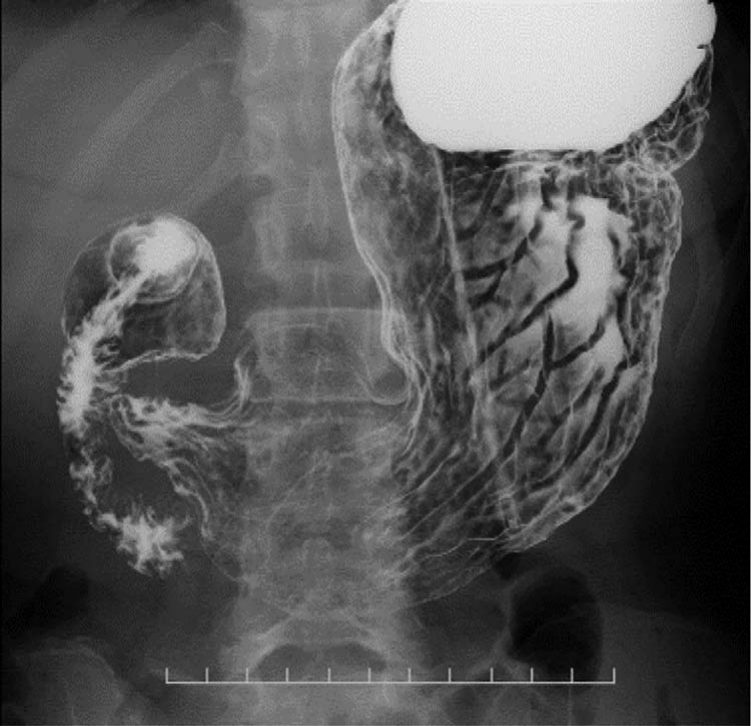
CT images showing a horizontal section of the esophagus; CT image shows circumferential wall thickening with infiltrative changes in the middle part of the thoracic esophagus.
